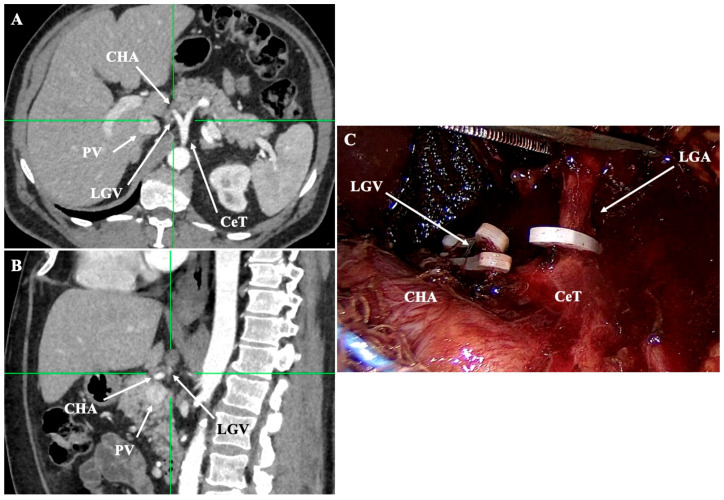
Preoperative CTA (A,B) and intraoperative view (C) demonstrating an LGV running posterior to the common hepatic artery (CHA) before draining into the portal vein (PV). This pattern (type I in Lee et al. [30] classification) is the most common LGV course and is clinically important during station-7 dissection, as early identification reduces the risk of avulsion. Personal archive of Assistant Professor Orestis Lyros.
